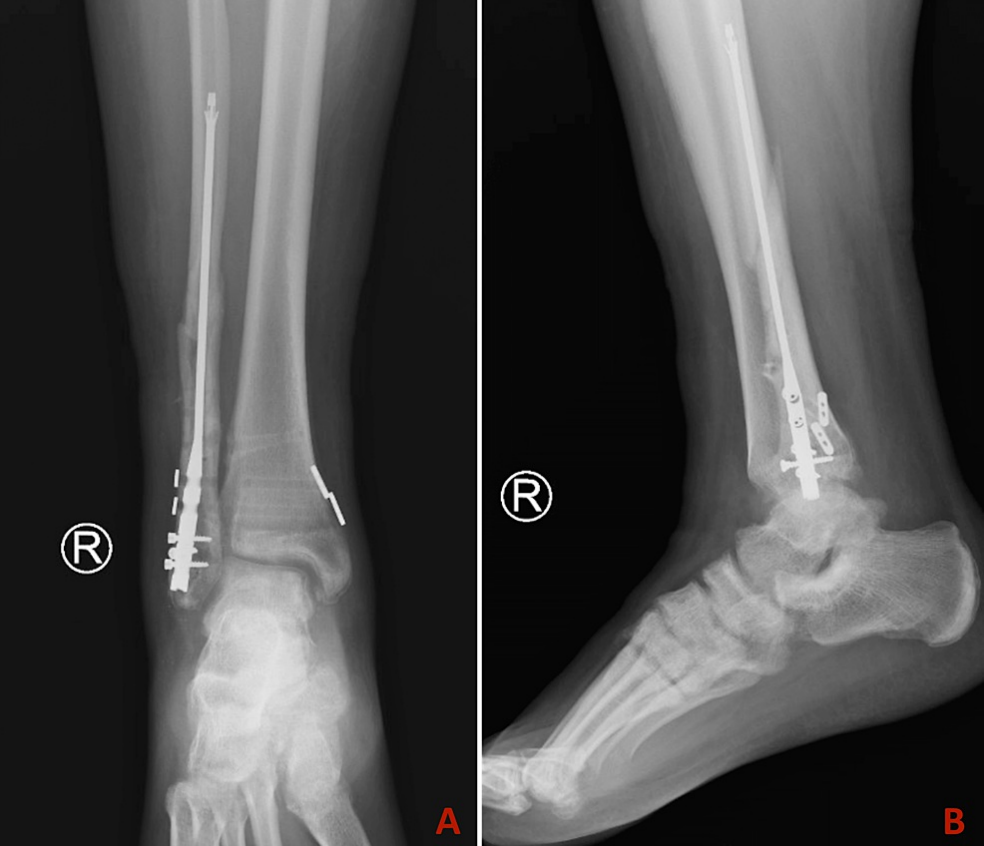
Postoperative radiographs at three-month follow-up. A: Anterior-posterior view. B: Lateral view
